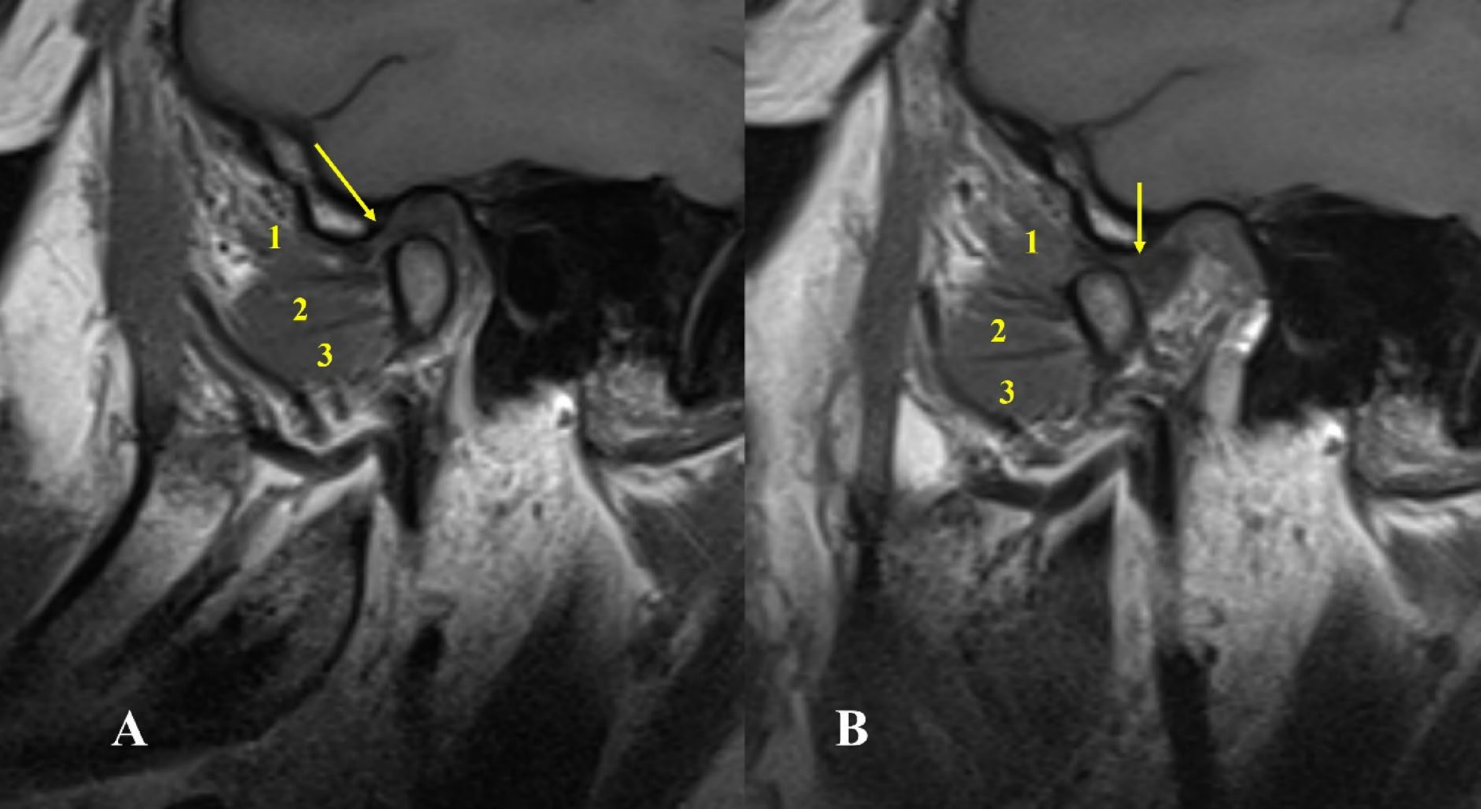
Oblique sagittal T1-weighted images represent Type III-B with three heads of lateral pterygoid muscle, the upper attached to the disc and condyle and the lower two attached to the condyle.
